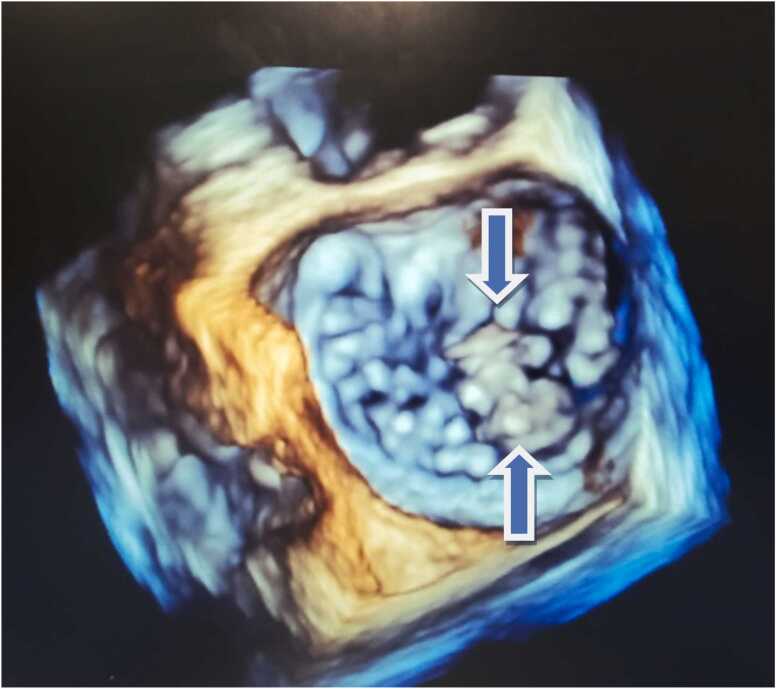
Three dimensional Echocardiography showing the mitral vegetation.
